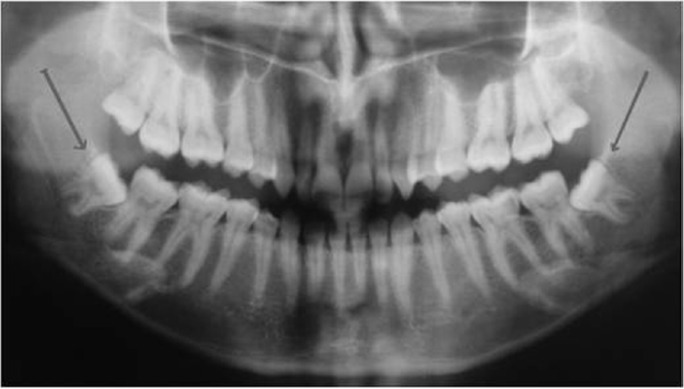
A panoramic roentgen of a patient (MD) operated for removal of mandibular third molars. The classifications of third molars were compatible with Class II, in Mesio-Angular position according to Archer Third Molar Classification.
